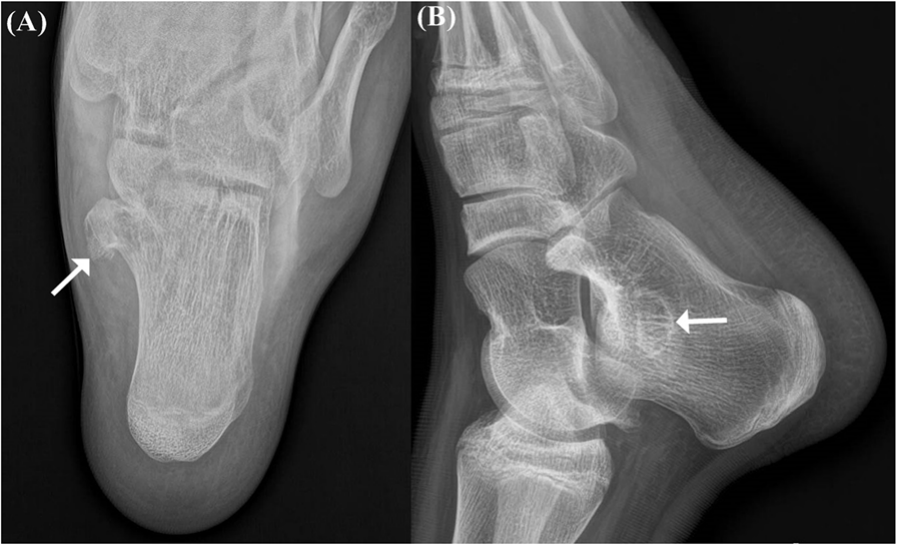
Preoperative axial (a) and lateral (b) plain radiographs of the calcaneus. A large peduculated mass is located posteroinferomedial to the sustentaculum tali. Corticomedullary continuity is observed between the mass and the sustentaculum tali (arrows)
